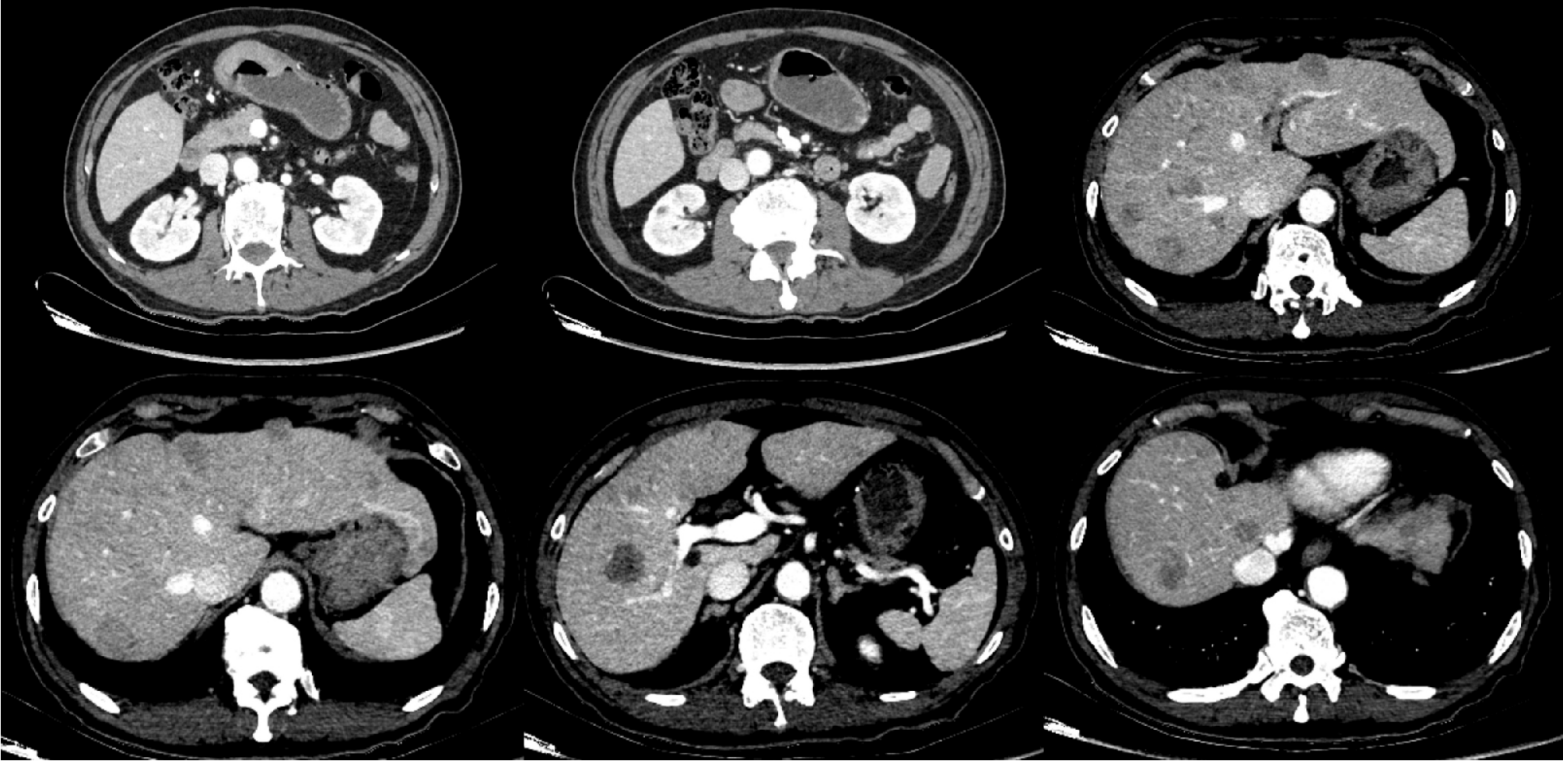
Pre-treatment CT scan demonstrating gastric antral wall thickening (findings suspicious for malignancy), retroperitoneal lymph node metastasis, and multiple liver metastases.
